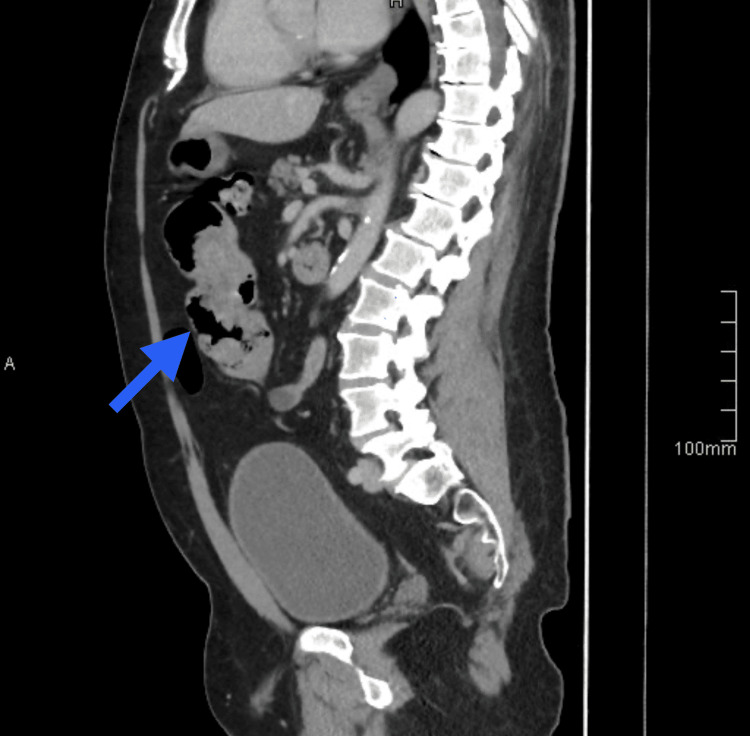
CT scan, sagittal view The arrow points to the intussuscepted segment.
